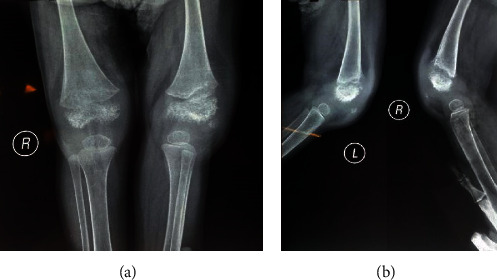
Knees X-ray shows metaphyseal irregularity, widening of growth palate, and soft tissue swelling (the first case).
